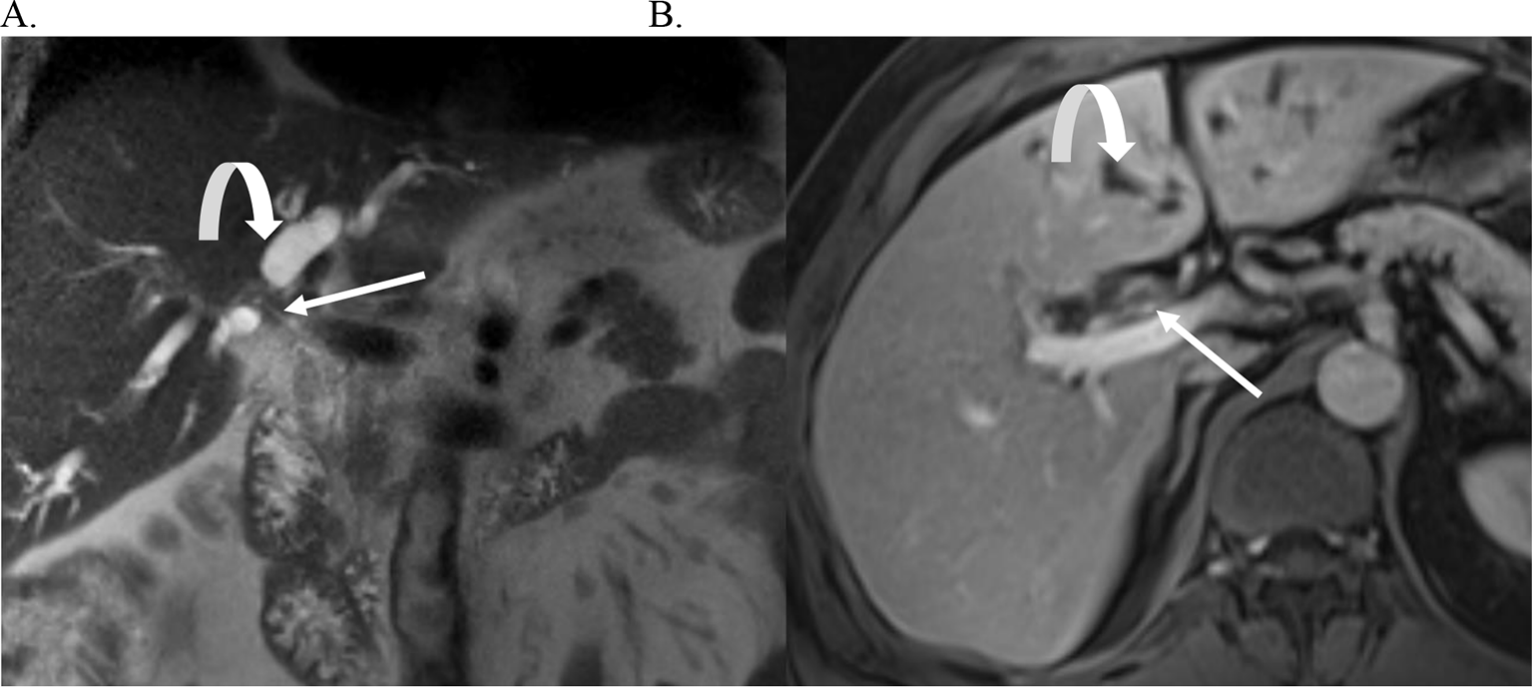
Perihilar Cholangiocarcinoma. Coronal T2-weighted image demonstrates a T2 dark stricture at the biliary hilum (arrow) (A). T1-weighted portal venous phase image demonstrates narrowing and wall enhancement of the common hepatic duct at the biliary hilum consistent with a periductal infiltrating perihilar CCA. There is upstream biliary ductal dilatation (curved arrows in A and B)
